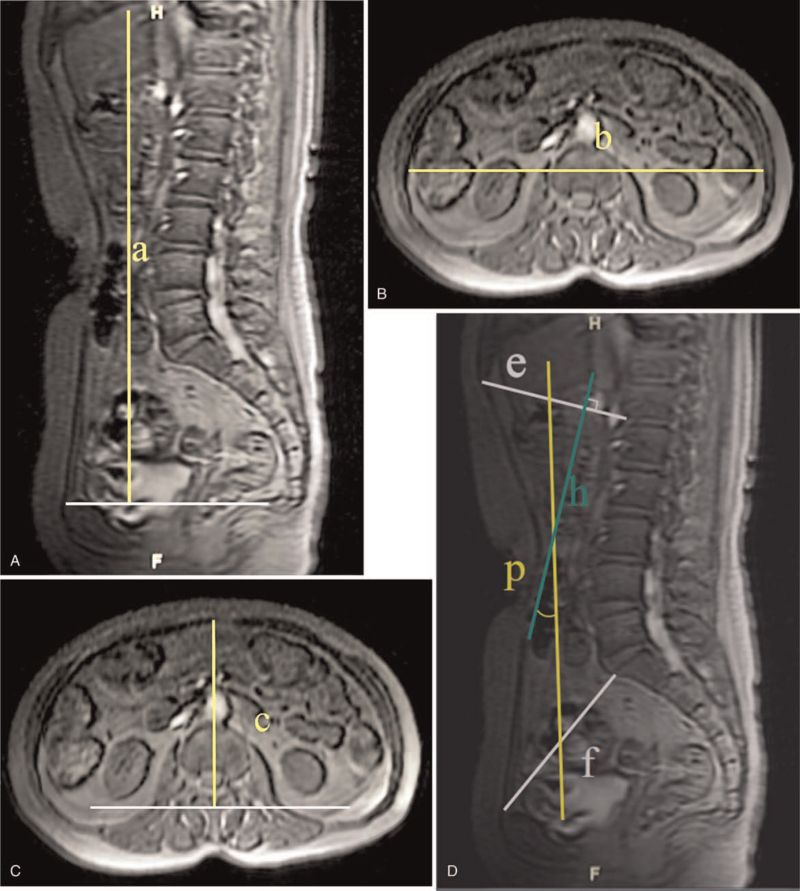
Measurements of ACV and DR on MRI. (A) Longitudinal diameters (a) of the abdominal cavity; (B) Transverse diameters (b) of the abdominal cavity; (C) Anterior-posterior diameters (c) of the abdominal cavity; (D) measurements of DR on MRI ACV = abdominal cavity volume, DR = diaphragm rotation.
